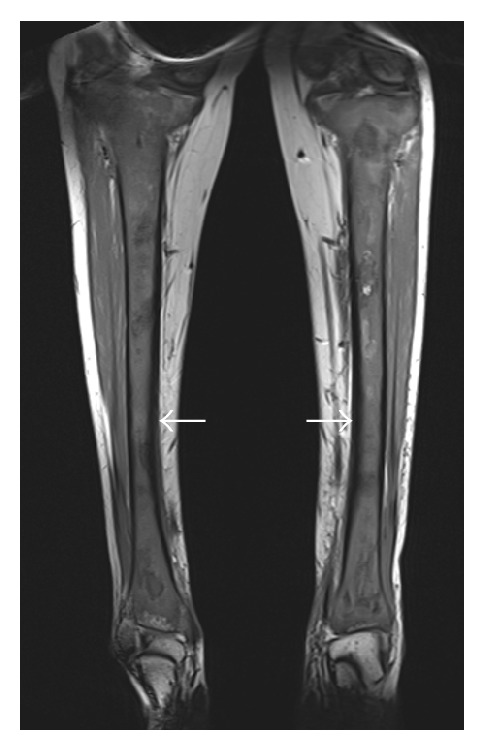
Bilateral tibial coronal T1 MRI showed bilateral asymmetrical hypointense lesions replacing the normal fatty signal intensity of both tibiae as compared to the normally appearing marrow fat signal in the talus. Note also the cortical thickening noted in the tibial cortices medially (arrows).
